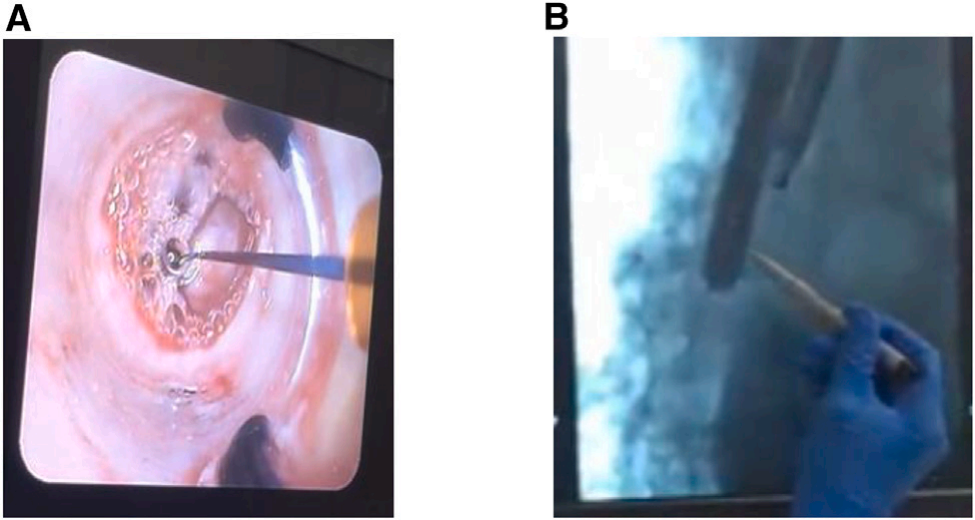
Endobronchial image screenshot from the teleproctor’s screen (A) This is an image of the endobronchial view from the bronchoscope camera as seen on the teleproctor’s screen. The teleproctor has a clear view of the entire bronchoscopy screen, including the patient’s airways, and the balloon and electrode of the catheter. This is the same view that is seen by the on-site treating physician team. Image not to scale. (B) Fluoroscopy image screenshot from the teleproctor’s screen. This is an image of the fluoroscopy view projected within the bronchoscopy suite and to the teleproctor. The X-ray of the patient’s chest is well visualized including the bronchoscope and the contrast-filled balloon within the esophagus. (Image not to scale. Image has been cropped to anonymize it).
